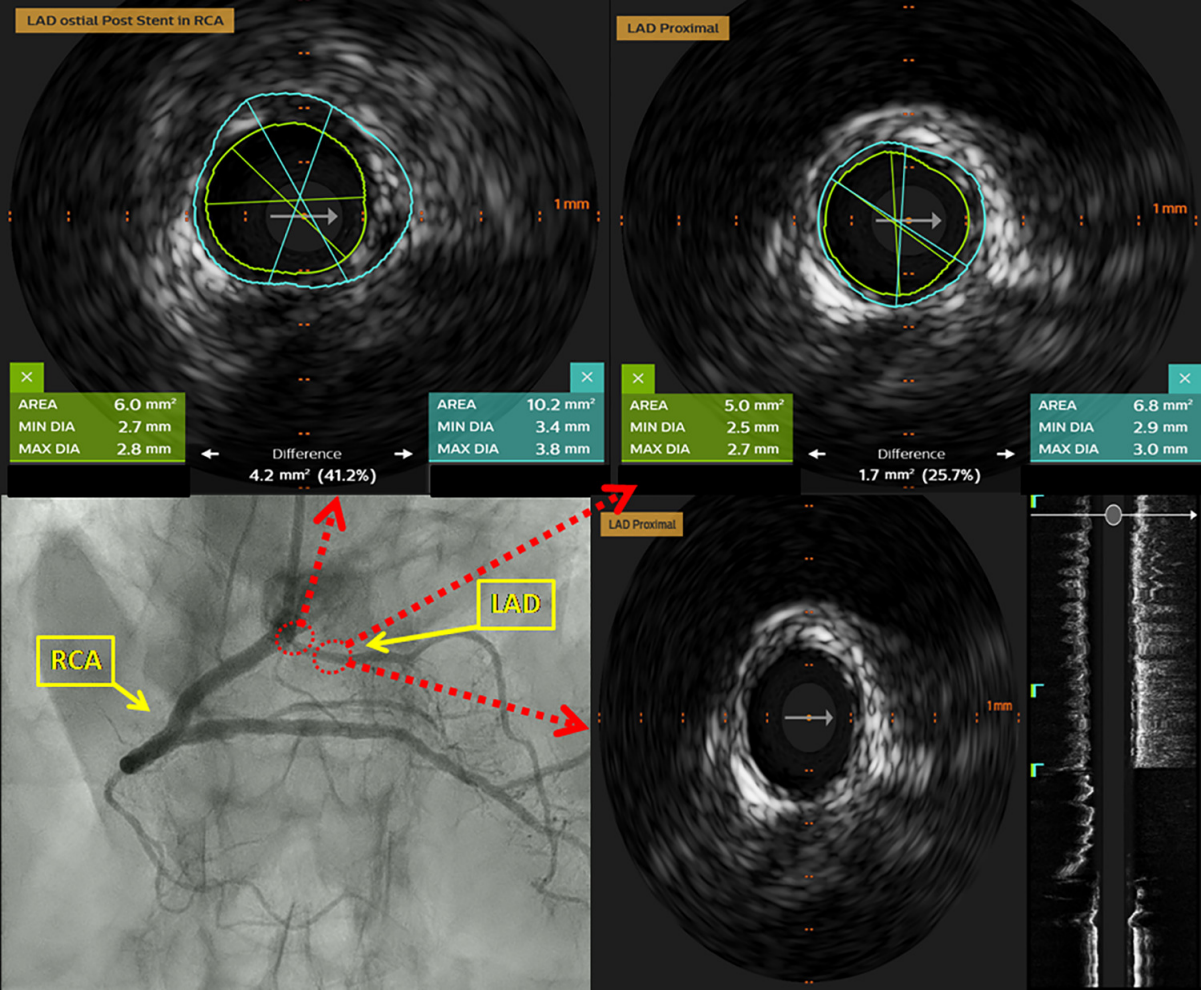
Intravascular ultrasound (IVUS) of the LAD after PCI. No signs of the narrowing of the ostial and proximal LAD after PCI of proximal RCA was documented.
